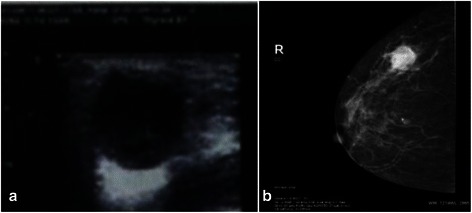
The imaging features of CNC. (a): Ultrasonography indicated a well-defined, heterogeneous, hypoechoic lesion. (b): The tumor was manifested as a irregular nodule with a heterogeneous, higher-density shadow, associated with tortuous vasculature around the tumor.
